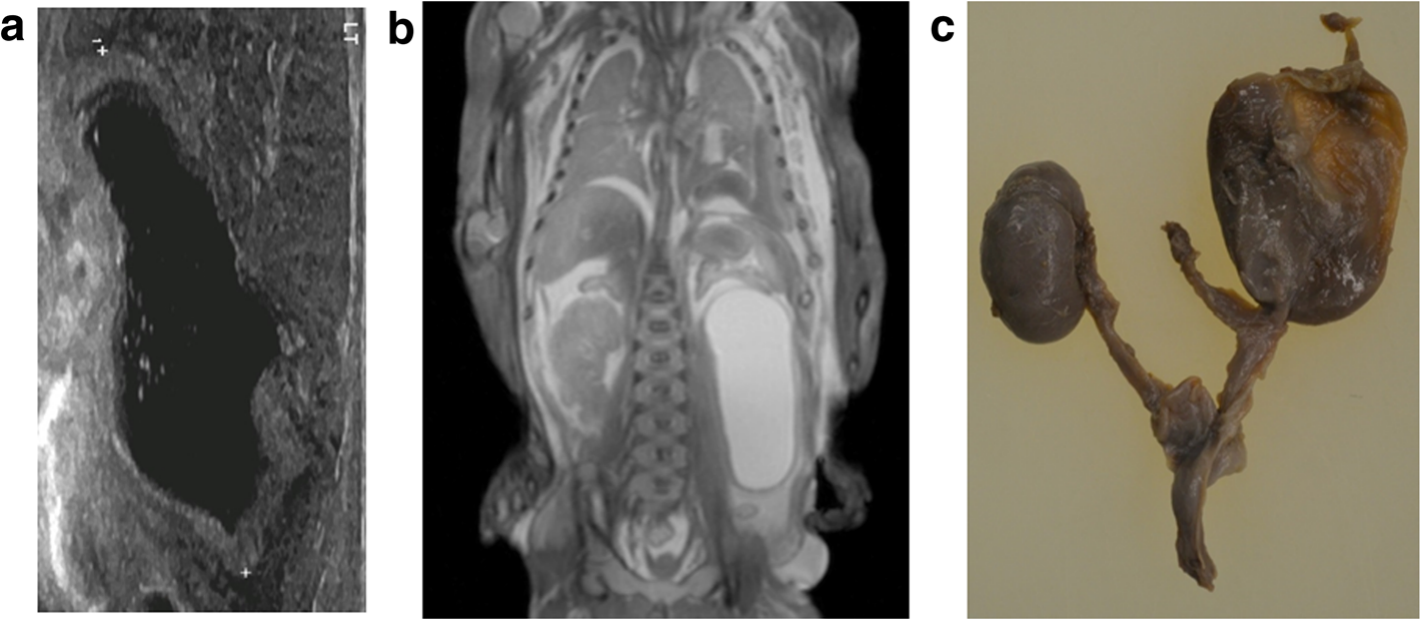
Unilateral left renal outflow obstruction from pelviureteric junction obstruction (PUJO) in a foetus at 21 weeks gestation, after termination of pregnancy. Post-mortem imaging was performed 10 days after delivery. The sagittal post-mortem ultrasound image of the left kidney (a) demonstrates a dilated renal pelvis, with only a thin rind of renal cortex stretched around the pelvis. The same finding is shown on the post-mortem T2-weighted MRI image in coronal plane (b), and a normal right kidney is demonstrated. A photograph of the extracted kidneys and ureters at autopsy (c) confirm the imaging findings of a much larger and dilated left kidney, with normal right kidney
